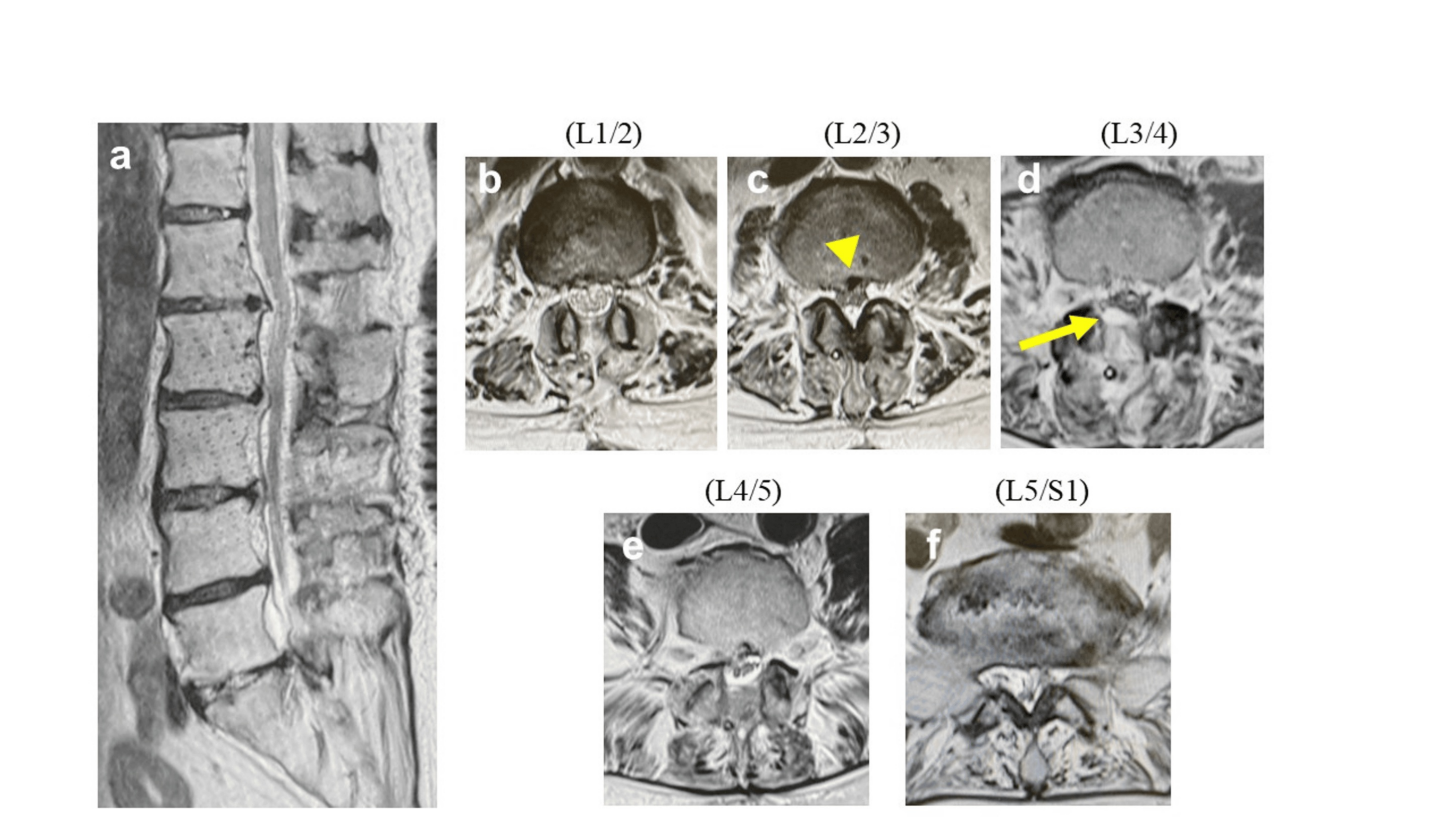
MRI on the second day after surgery (a) Sagittal image of lumbar spine. The spinal canal was decompressed at L1/2, L3/4, and L4/5. (b) Axial image of L1/2. (c) Axial image of L2/3. Cauda equina consolidation at the L2/3 level was shown (yellow arrowhead), which was not operated on. (d) An axial image of L3/4. The right-leaning epidural hematoma of L3/4 was shown (yellow arrow). (e) Axial image of L4/5. (f) Axial image of L5/S1.
